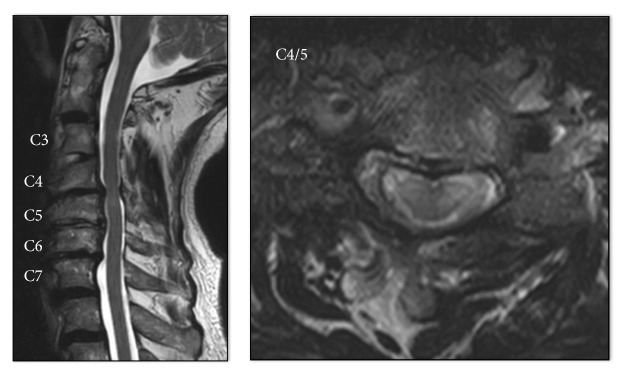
Magnetic resonance image showing C3–C7 degenerative spondylosis with mild central canal stenosis and high-signal lesions at C4/5.
